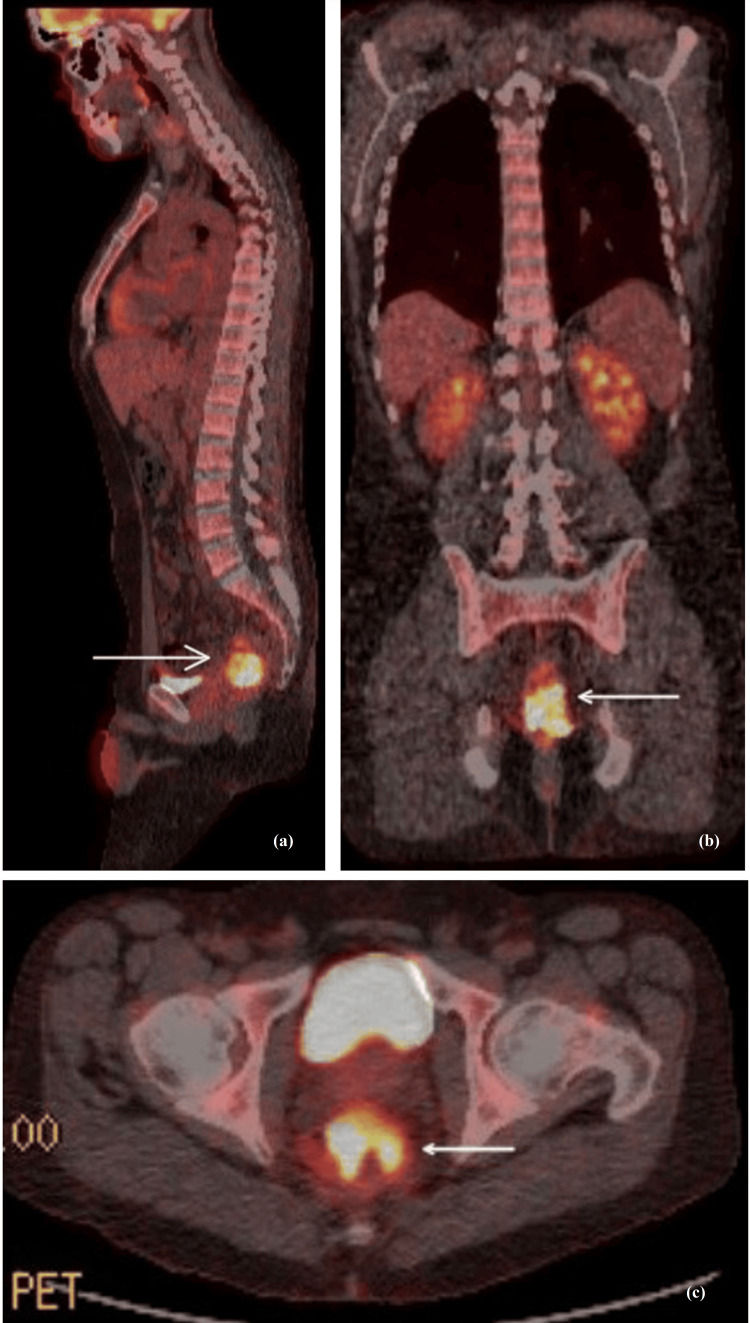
PET-CT scan images Positron Emission Tomography-Computed Tomography imaging (PET-CT) scan images show a primary neoplastic rectal mass with SUV of 16.5 and multiple centimeters and subcentimeter presacral lymph nodes without associated FDG uptake. (a) Sagittal view (b) Coronal view (c) Axial view
